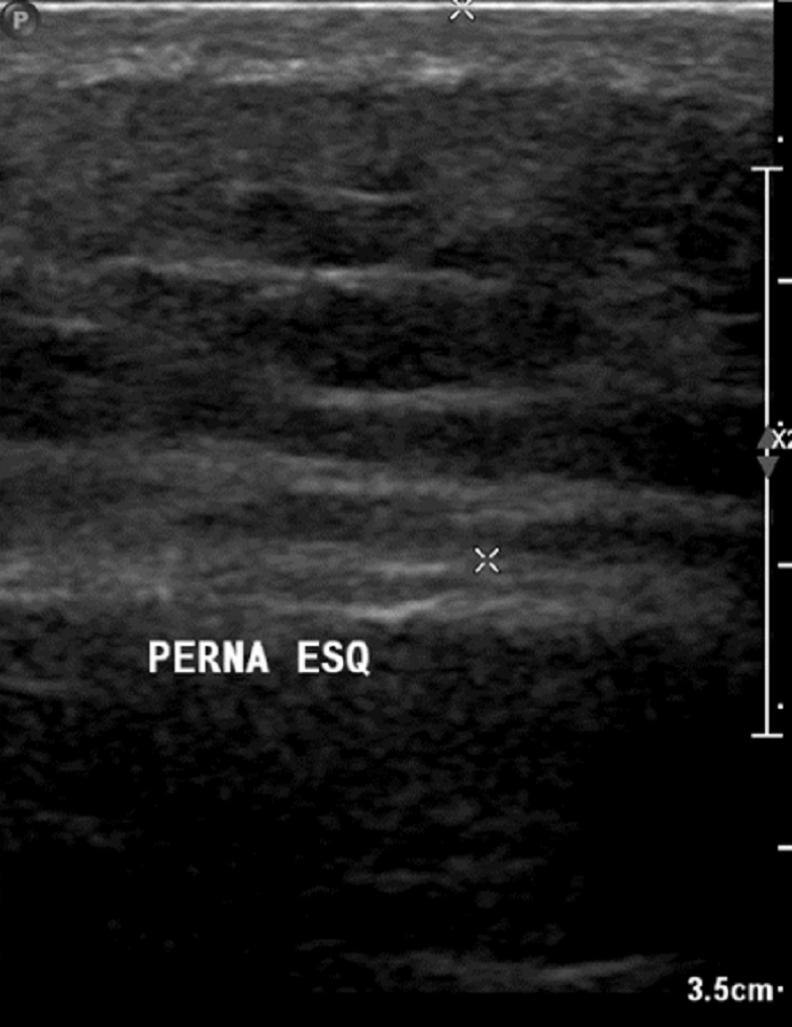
Post-treatment ultrasound showing the onset of the reconstruction of the skin layers
